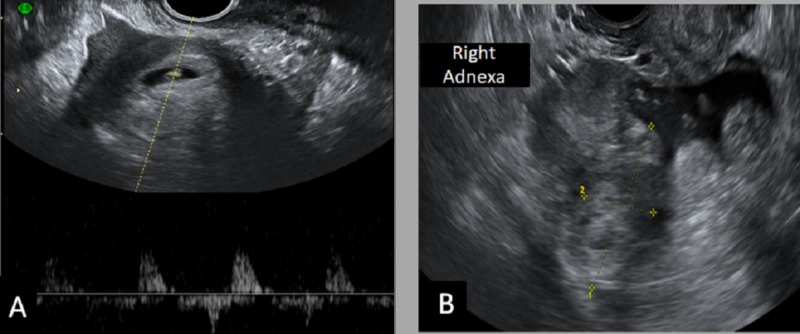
Transvaginal ultrasound A) The viable intrauterine gestational sac is seen with the fetal heartbeat; B) a right adnexal mass lesion with surrounding hematoma is noted as well, indicative of heterotopic pregnancy.
